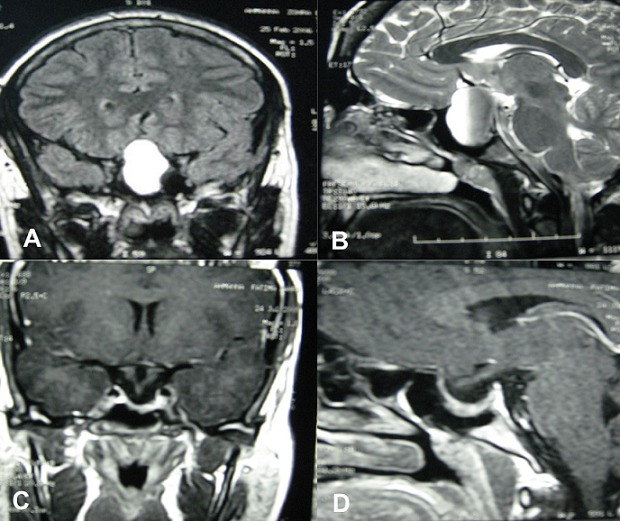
The magnetic resonance imaging (MRI) was in favour of a stroke of the pituitary macro-adenoma(A and B). The MRI of control shows the total disappearance of the leaving expansive process place in an intracellair arachnoidocèle (C and D)
